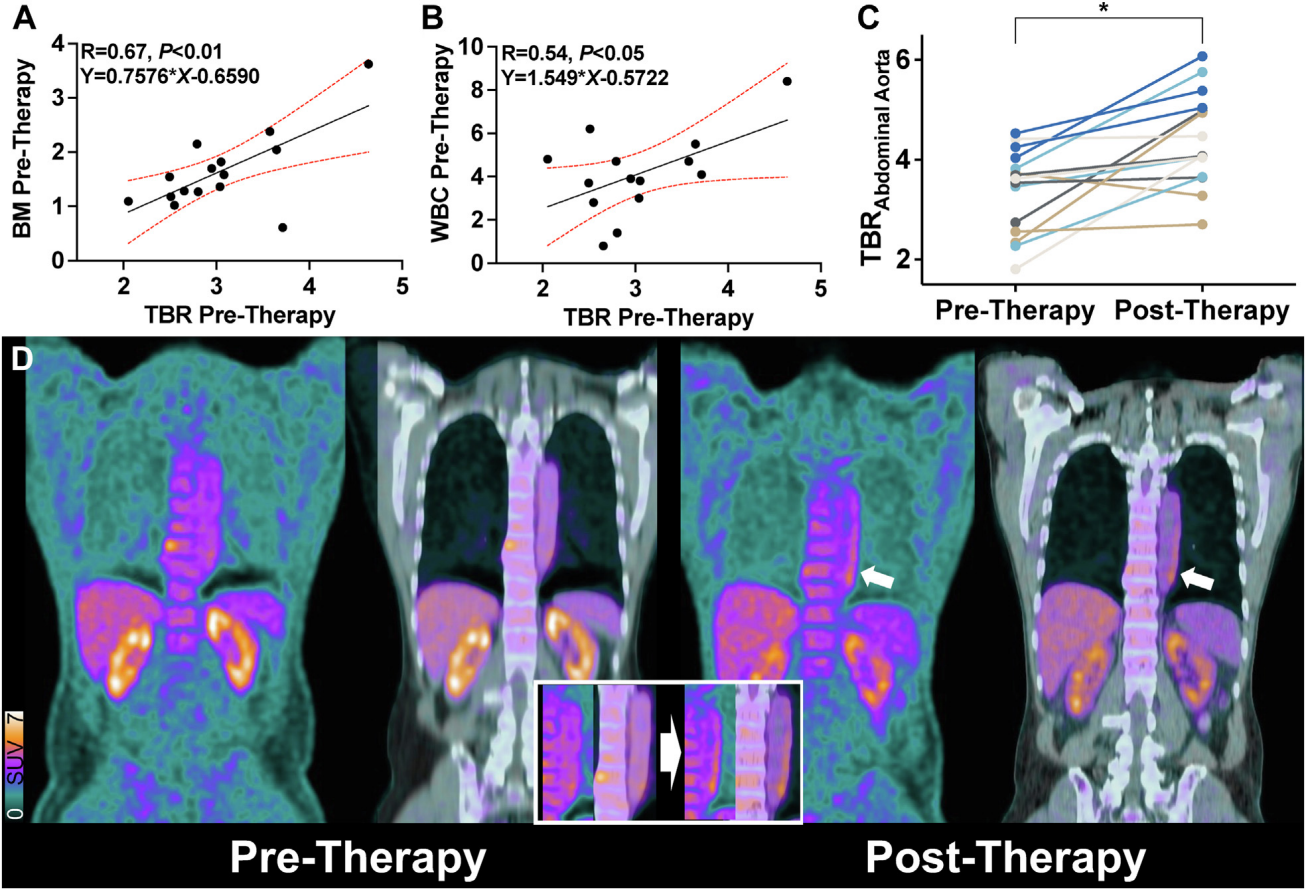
Inflammatory-Targeted PET Imaging After Transplantation (A) Pretherapeutic uptake derived from bone marrow (BM) and (B) white blood cells (WBCs) correlates with pretherapeutic target-to-background ratios (TBRs) derived from all vessel segments (WBC value missing in 1 subject). (C) Posttransplant change of TBR (as derived from abdominal aorta) demonstrates an increase in the majority of the subjects. (D) Coronal views of positron emission tomography (PET) and PET/computed tomography of the thoracic aorta prior to (left) and after (right) allogeneic stem cell transplantation. Three months after therapy, vessel wall uptake is markedly increased (white arrows, inset). ∗*P* = 0.001.
